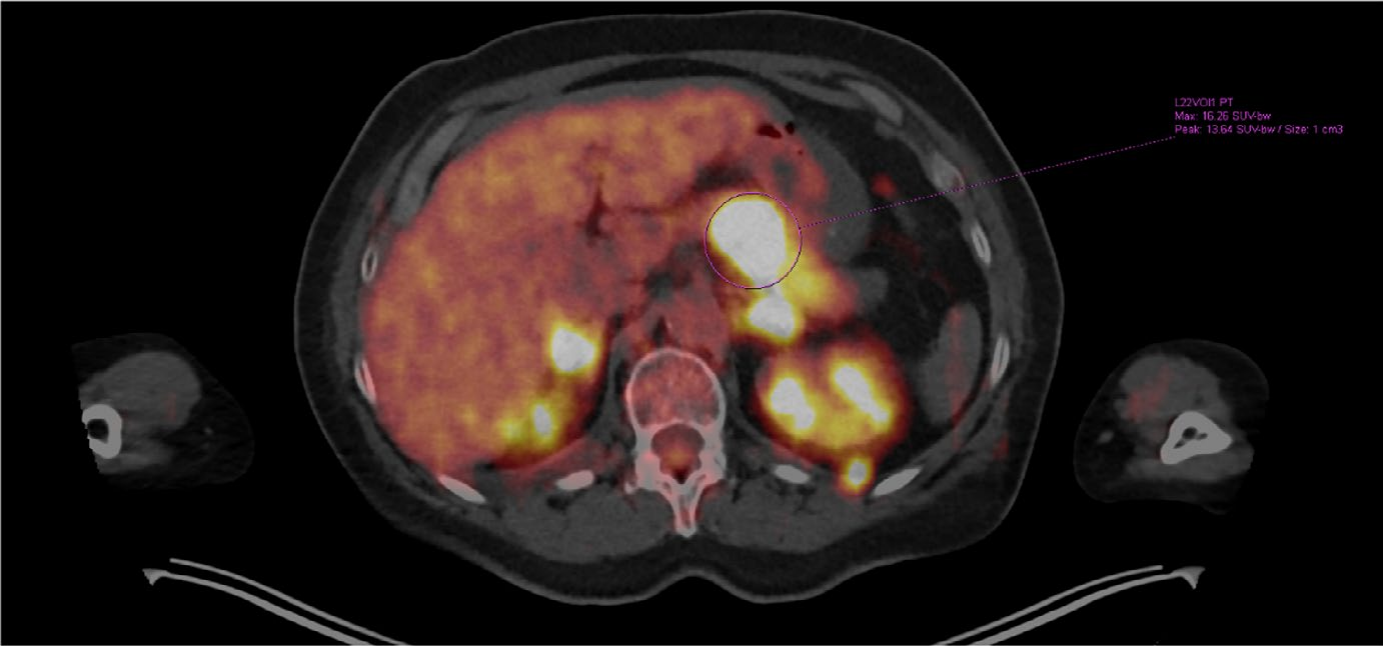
PET/CT whole body survey, transverse image, showing increased FDG avidity of adrenal glands, numerous metabolically active renal lesions, and FDG avid mass within the body of the pancreas
